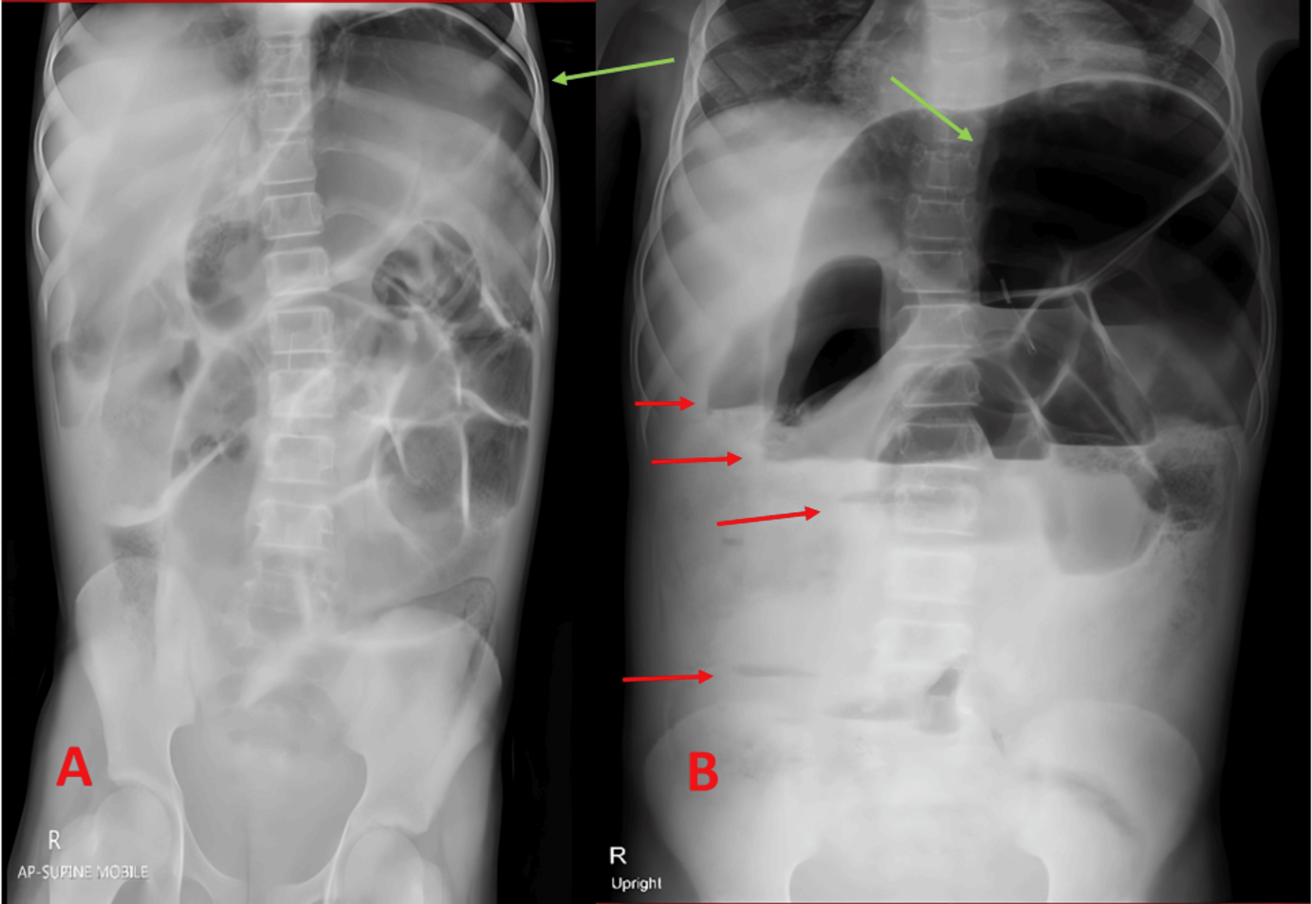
Early Abdominal Radiographs (Acute Distension) (A) Supine AP abdominal radiograph showing widespread dilatation (green arrows) and air-fluid levels (red arrows), (B) Upright abdominal radiograph confirming large air-fluid levels (red arrows) without evidence of a mechanical transition zone.
